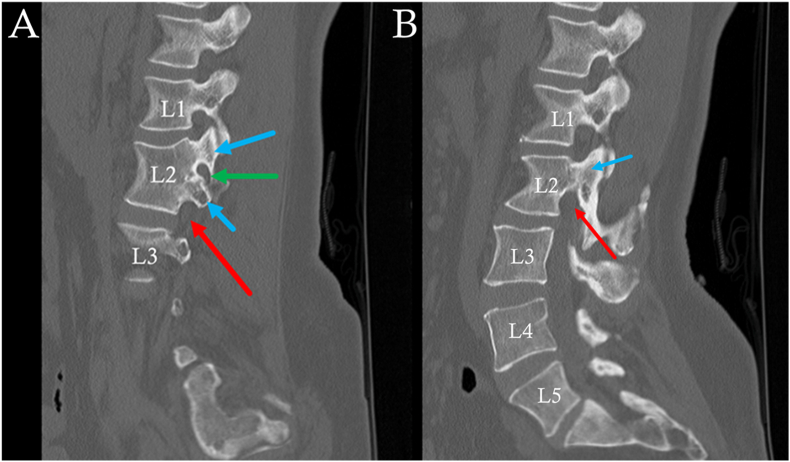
Sagittal CT Bone images of right (A) and left (B) lumbar spine. Appreciate multiple pedicles (blue arrows) on the right, and single pedicle on the left. Corresponding “pseudo foramen” (green arrow) compared to typical foramen (red arrows). (For interpretation of the references to colour in this figure legend, the reader is referred to the Web version of this article.)
